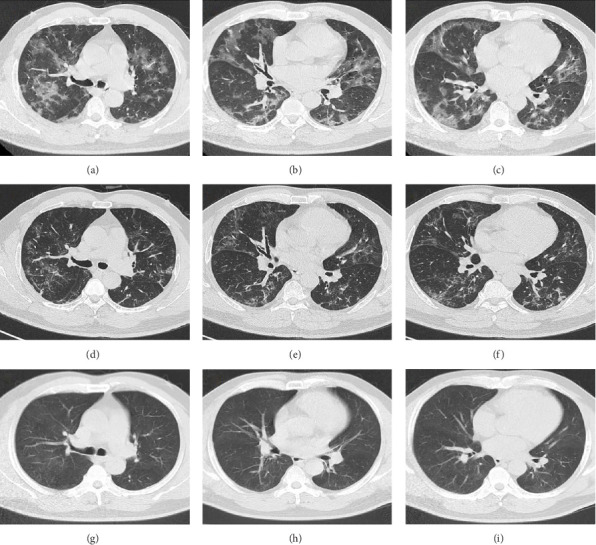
Time course of the patient's chest computed tomography findings (a–c, at admission; d–f, 30 days after admission; g–i, 3 months after admission). The ground glass opacities in both lungs indicate COVID-19 (a–c). The final chest CT showed improvement in lung lesions after treatment (d–f and g–i).
